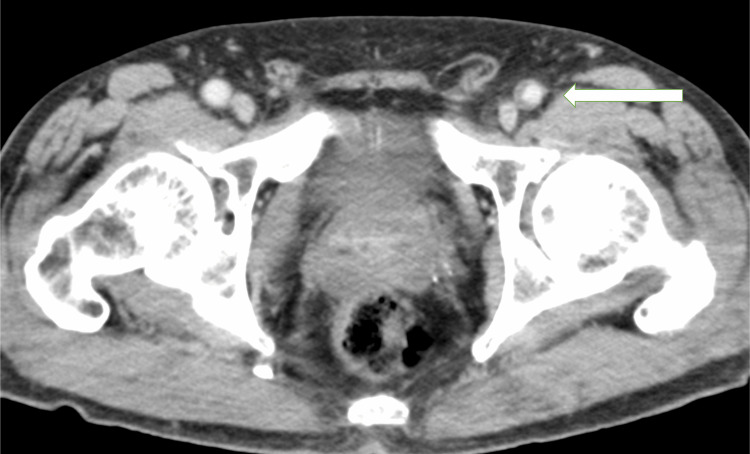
The previous CT image performed at our hospital Left CFA is not aneurysmal, but a mural thrombus is observed. A timer is thought to have developed in the mural thrombus and rapidly formed an aneurysm (⇦). CT - computed tomography; CFA - common femoral artery
